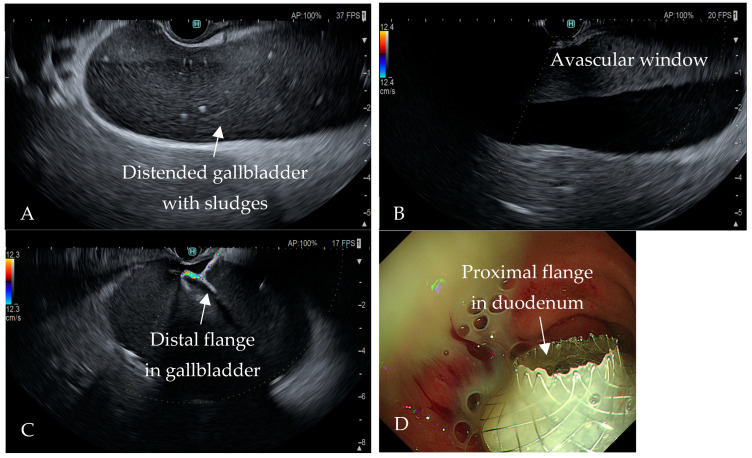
Representative steps of a lumen-apposing metal stent (LAMS)-based endoscopic ultrasound-guided gallbladder drainage (EUS-GBD) (A) EUS image showing a distended gallbladder with sludge adjacent to the duodenum. (B) Color Doppler confirmation of an avascular access window before cautery-enhanced access. (C) Deployment of the distal flange of the LAMS under EUS guidance. (D) Endoscopic view showing bile or purulent drainage through the deployed LAMS.
